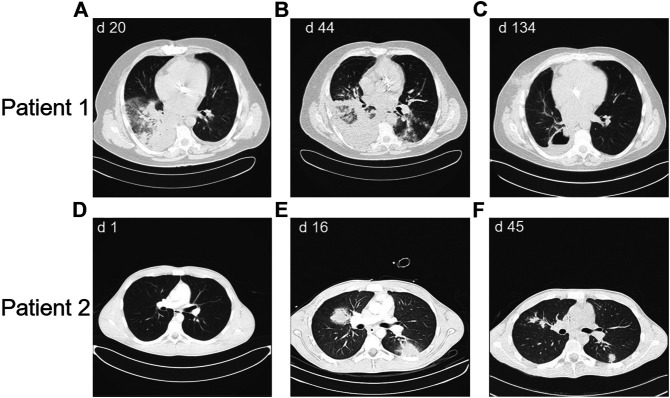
Thoracic CT scans showing the course of mycotic lesions in Patient 1 (panels A-C) and patient 2 (panels D-F), d = day after diagnosis of ALL.
